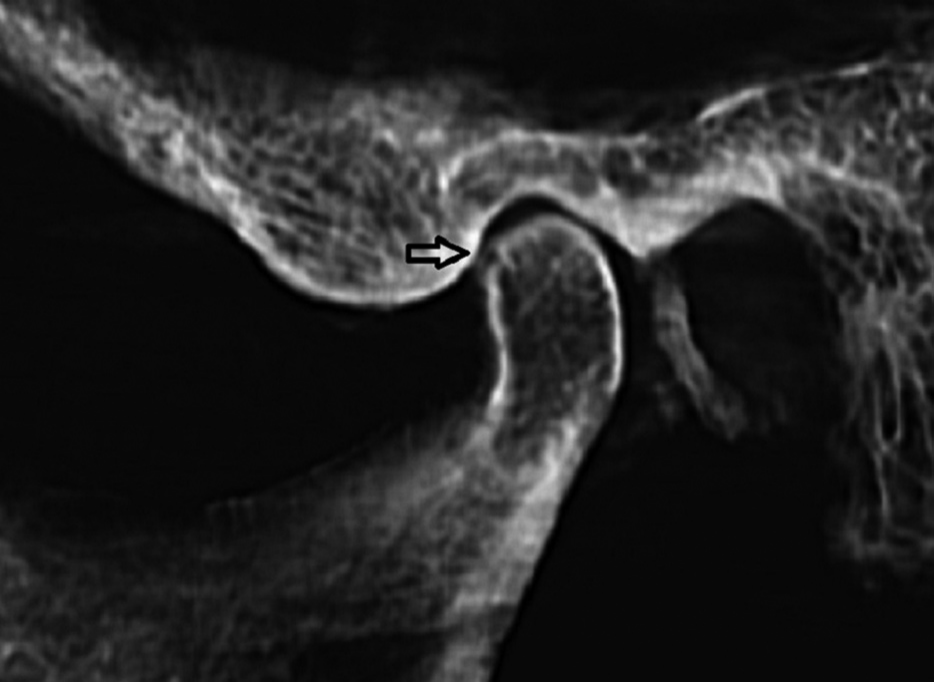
CBCT image shows sagittal view of an erosive lesion on condylar head.
